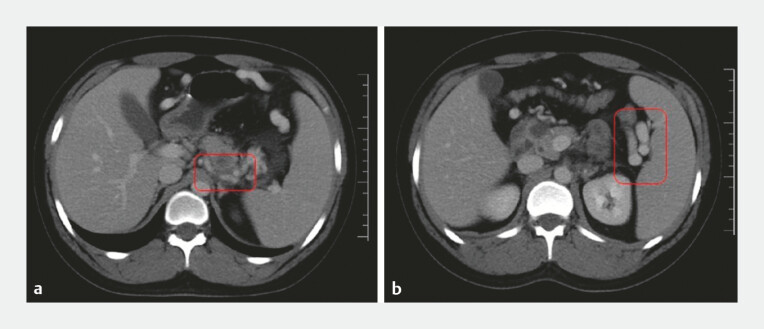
Contrast-enhanced computed tomography showing
